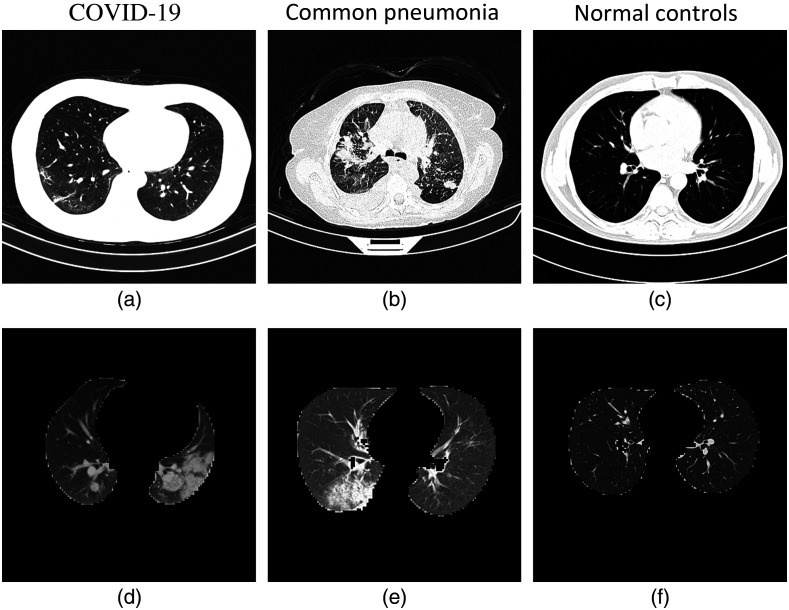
Typical transverse-section CT images: (a) complete CT images and (b) segmented CT images.
